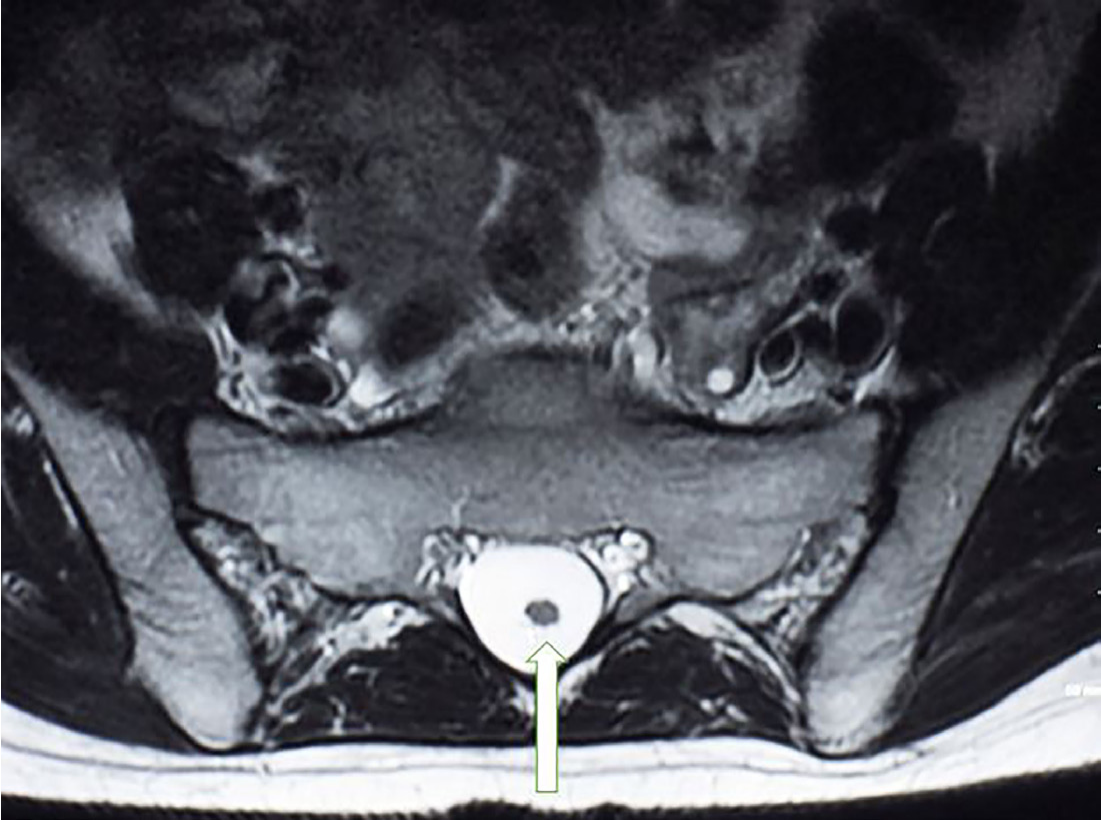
Axial view of the T2-weighted magnetic resonance image showing the continuation of the spinal cord into the sacral region (arrow).
